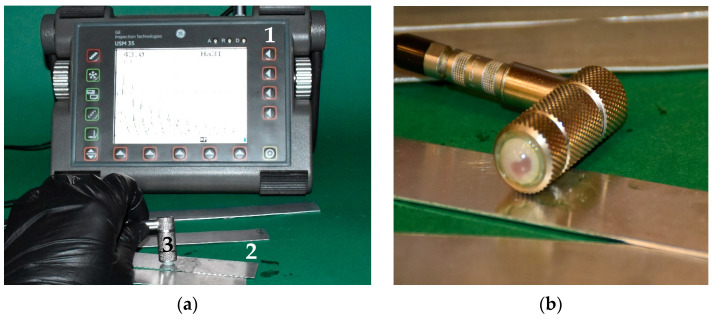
Measuring system: (a) view of the test station during testing, 1—ultrasonic flaw detector, 2—test sample, 3—ultrasonic probe; (b) ultrasonic transducer with water delay line.
